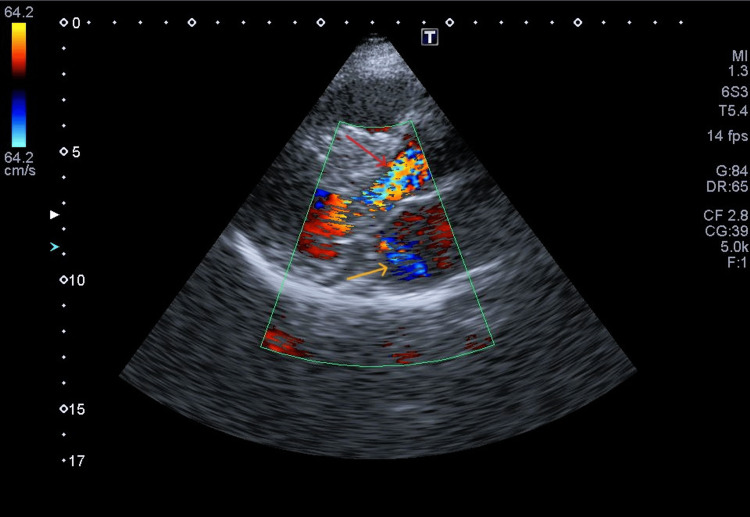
Dynamic left ventricular outflow tract obstruction and mitral regurgitation Parasternal long-axis color Doppler transthoracic echocardiographic view demonstrating turbulent systolic flow across the left ventricular outflow tract consistent with dynamic obstruction (red arrow), along with a posteriorly directed mitral regurgitation jet (yellow arrow).
